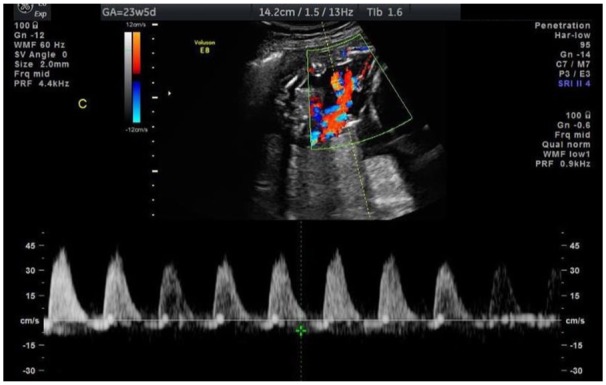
Umbilical artery Doppler ultrasound at 23w5d revealing reversed diastolic flow in Fetus C.
